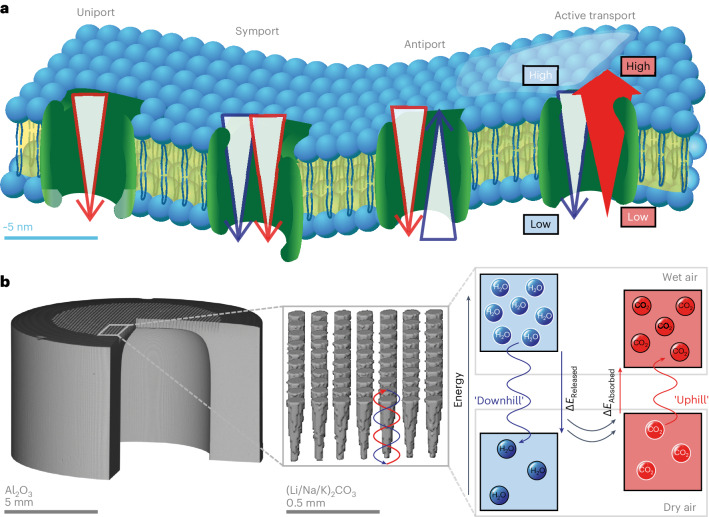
Transport in biological membranes and the humidity-driven synthetic membrane. a, In biological membranes, transport is typically passive, down a concentration gradient (uniport, symport and antiport); however, in active transport, uphill transport against a concentration gradient for one species (red) can be achieved via an intimate coupling with downhill transport of a second species (blue). b, A three-dimensional reconstruction of micro-computed X-ray tomography scans of a synthetic, supported molten-salt membrane, comprising an alumina (Al_2_O_3_) support with a ternary eutectic mixture of molten carbonates ((Li/Na/K)_2_CO_3_) held in laser-drilled artificial pores. A humidity difference (wet air and dry air) is harnessed to pump CO_2_ from one air stream to the other, against its concentration gradient, to produce a CO_2_-enriched output stream.
